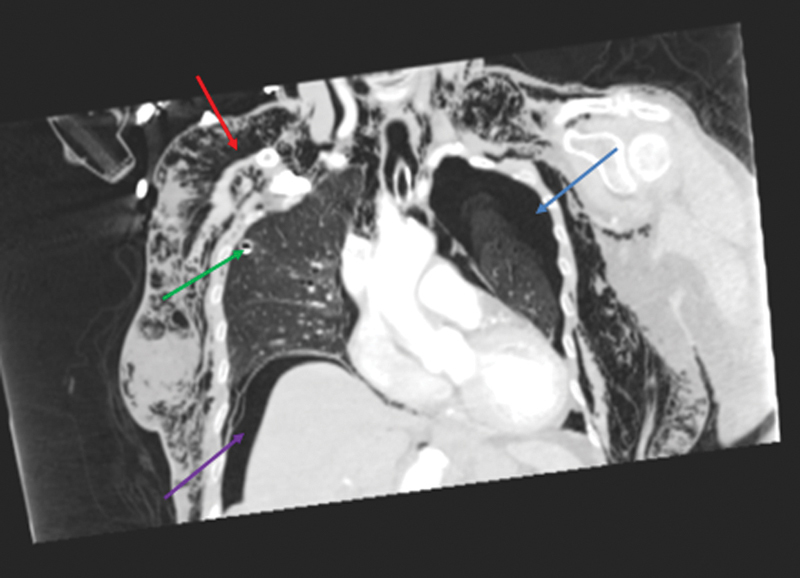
Coronal computed tomography image of extensive subcutaneous emphysema in the neck and thoracic wall (red arrow), left pneumothorax (blue arrow), and pneumoperitoneum (purple arrow). Part of the right chest drain is visible (green arrow).
